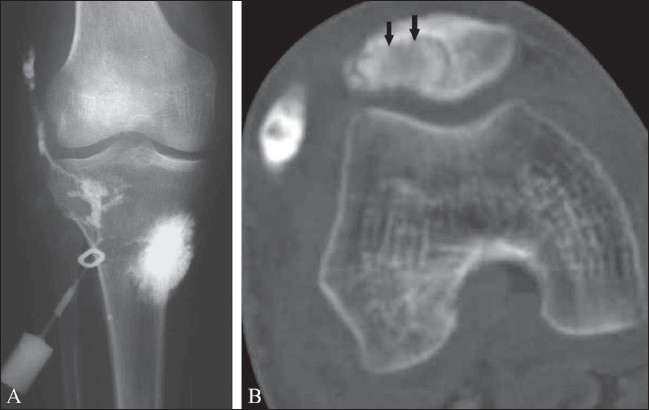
Tuberculous arthritis with fistula formation and chronic osteomyelitis of the patella. Frontal radiograph of the knee after a sinogram (A) shows sinus tract formation. CT scan after a sinogram (B) demonstrates contrast filling of a para-articular collection and a sequestrum within an osteolytic lesion in the patella (black arrows)
